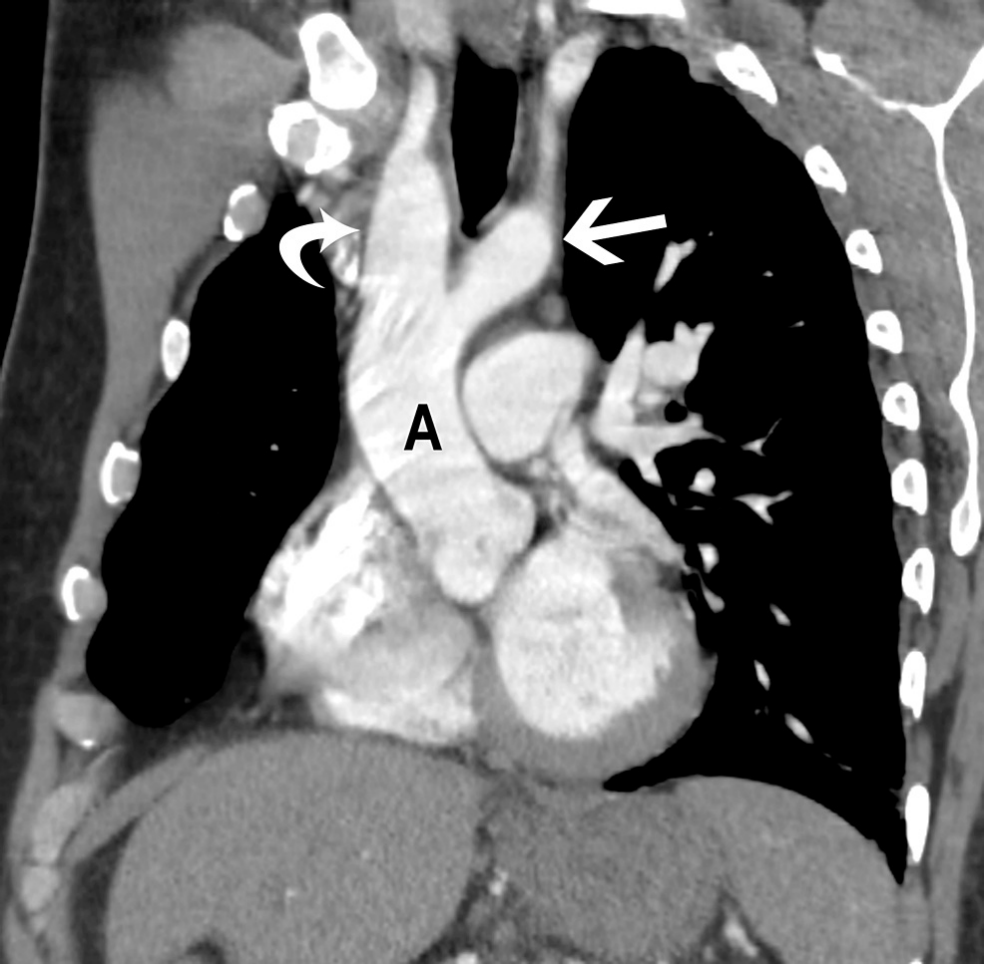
Coronal reformatted image from contrast-enhanced computed tomography scan of the chest shows the ascending aorta (A) splitting into the right (curved arrow) and left (straight arrow) aortic arches.
